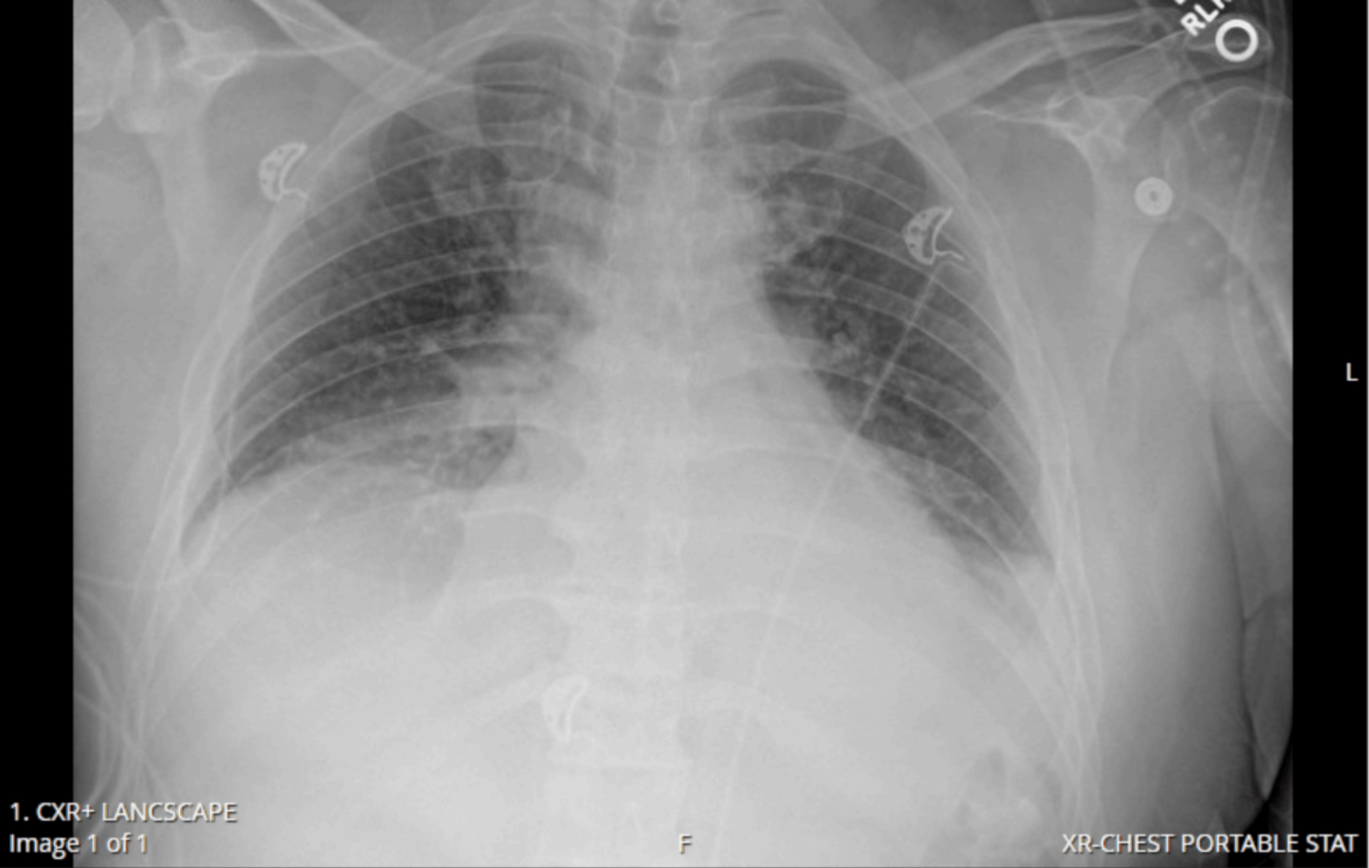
Chest x-ray just prior to intubation, revealing small bilateral pleural effusions with bibasilar airspace disease.
